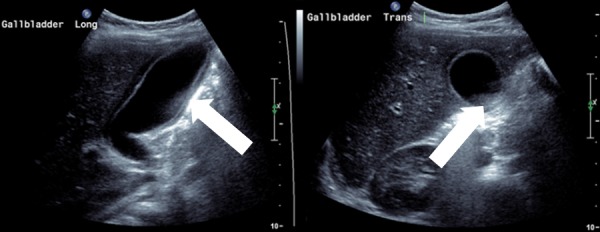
Ultrasound gallbladder – diffuse gallbladder wall thickening with pericholecystic collection. No intraluminal sludge or calculi seen.
